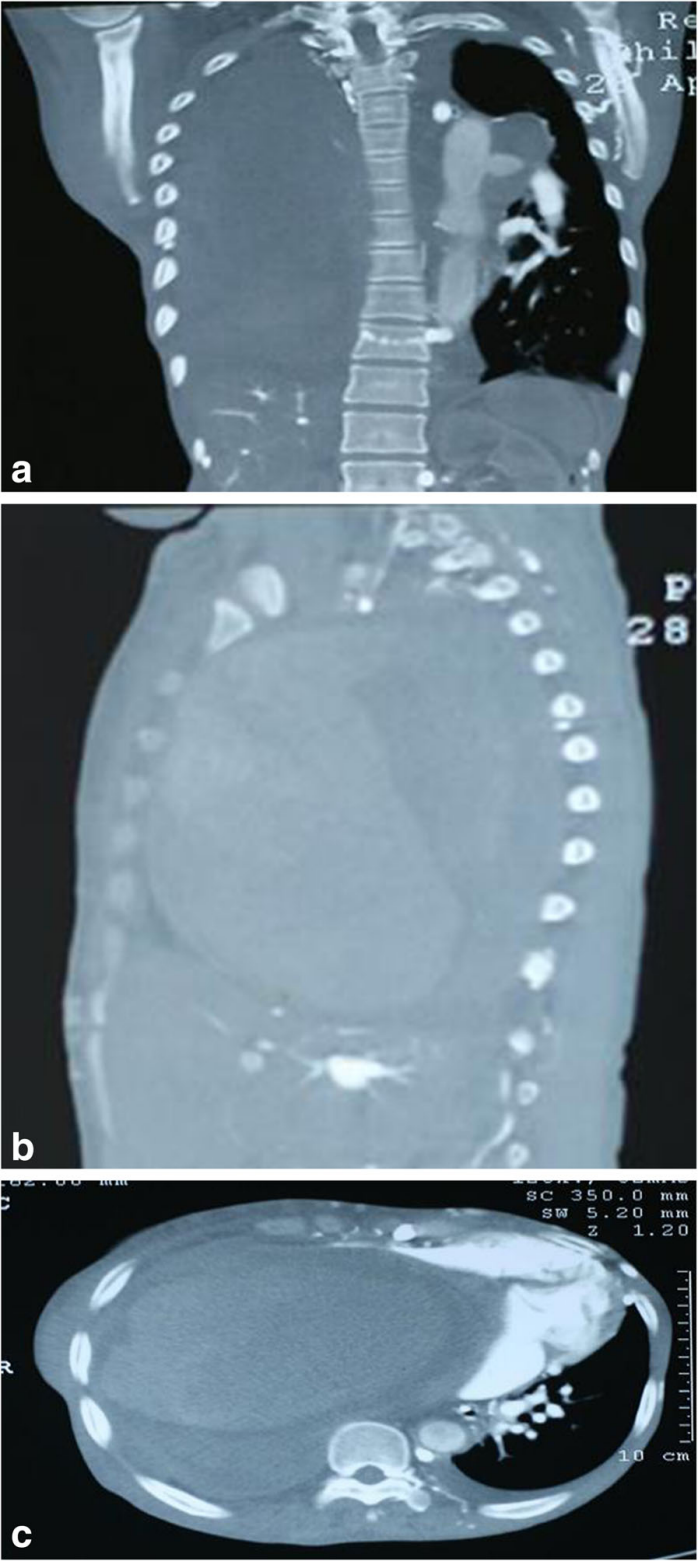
a Computed tomography chest (coronal view) displaying a huge right-sided mass. b Computed tomography chest (right parasagittal view) displaying a huge mass. c Computed tomography chest (axial view) displaying a huge right-sided chest mass causing mediastinal shift to the contralateral side
